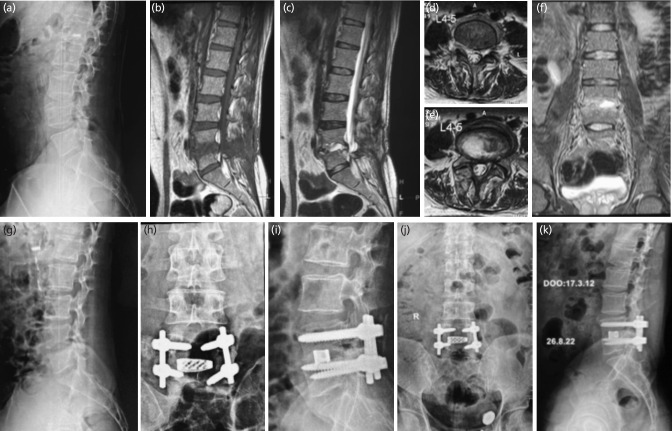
Male 22 years, operated micro lumbar discectomy for lumbar disc herniation L4-5. (a) Lateral radiograph before index surgery. (b-f) MRI T1-T2 sagittal, T2 axial and coronal sequences showing intra-discal abscess, left para-discal foraminal abscess, with end-plate destruction. (g) Lateral radiograph showing evident disc space narrowing and irregular end plate. (h, i) Antero-posterior and lateral radiograph showing transforaminal lumbar inter-body fusion executed with bone graft and cage with pedicle screws. (j, k) 95 months final follow-up antero-posterior and lateral radiographs showing stable united reconstruction.
